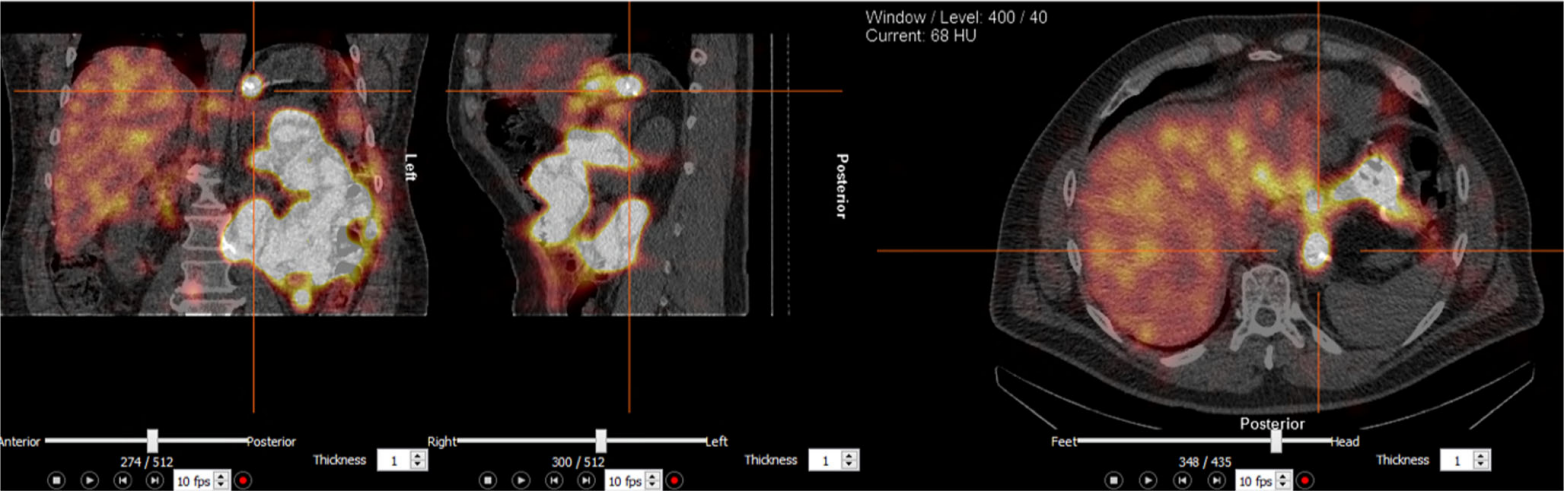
SPECT–CT scan at the end of bile reflux scintigraphy of a representative patient. Bile tracer activity in the gastric pouch and esophagus are shown
